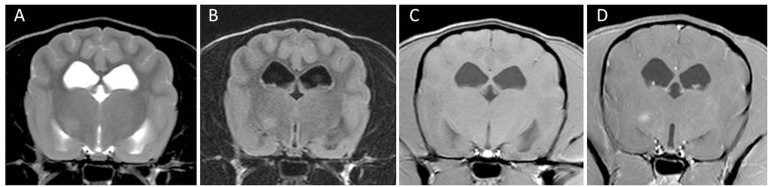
Transverse magnetic resonance images (3 T) of a Chihuahua (5 years, female) with necrotizing meningoencephalitis. Single T2 (A) and fluid-attenuated inversion recovery (B) hyperintense round-shaped lesion in the right diencephalon. There is a small uniform contrast enhancement in the center of the lesion on the post-contrast T1-weighted image (D) compared to the pre-contrast T1-weighted image (C).
